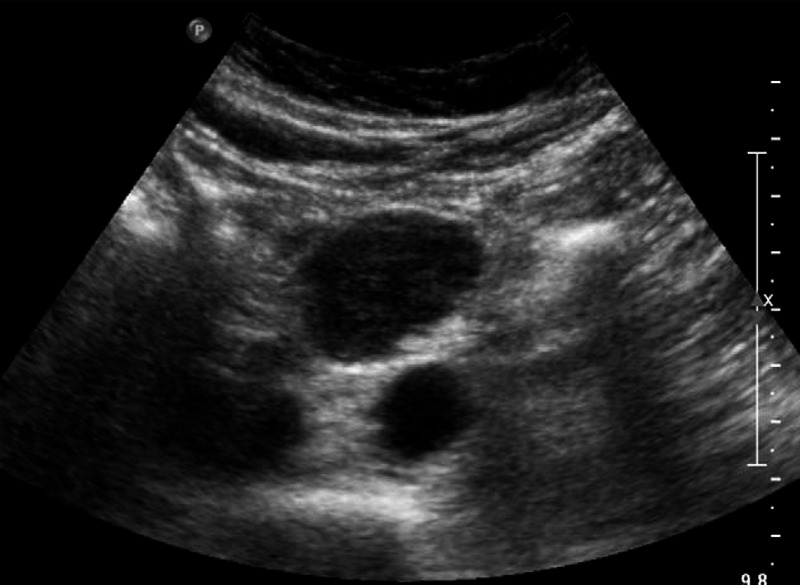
Abdominal ultrasound scan demonstrates an ovoid homogenous hypoechoeic mass anterior to the inferior vena cava and aorta in the mid abdomen.
